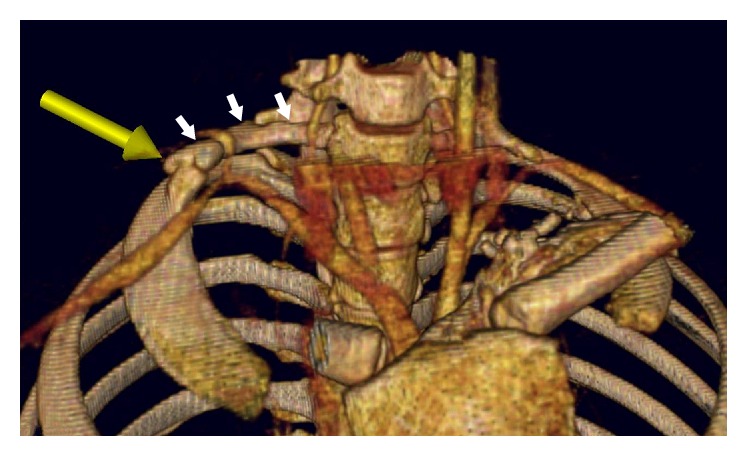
Three-dimensional volume rendering (VR) chest multidetector computed tomography (MDCT) demonstrating unilateral cervical rib (white arrows) and pseudoarthrosis (yellow arrow). The course of the subclavian artery is changed by unusual topographic relations.
